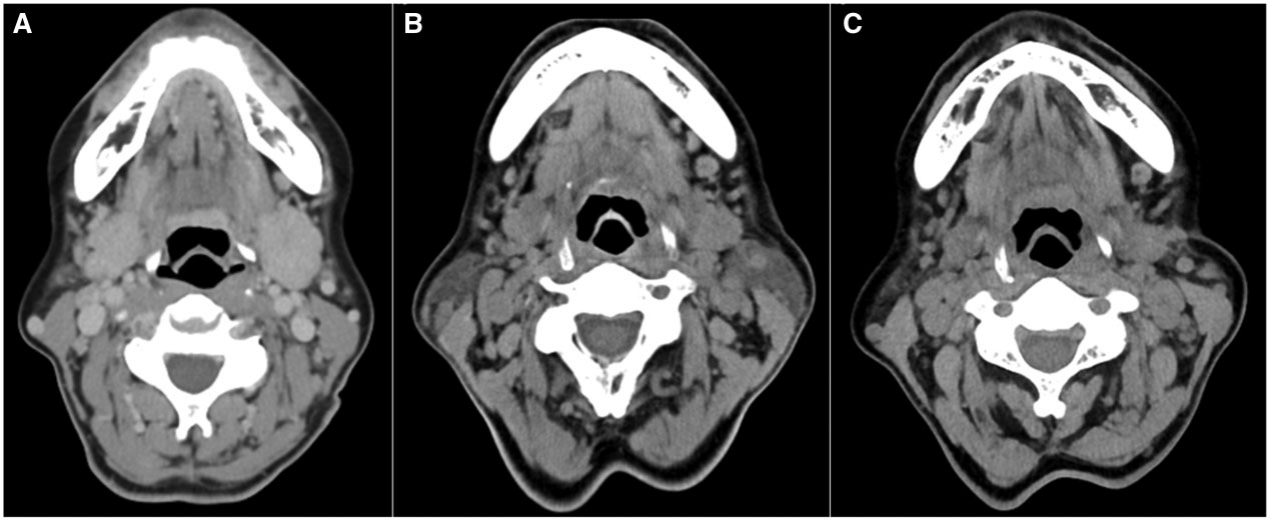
CT findings of submandibular glands before and after dupilumab therapy. CT revealed bilateral enlargement of submandibular glands at first visit (A), its reduction at 2 months after initiating dupilumab monotherapy (B), and complete resolution 7 months later (C)
